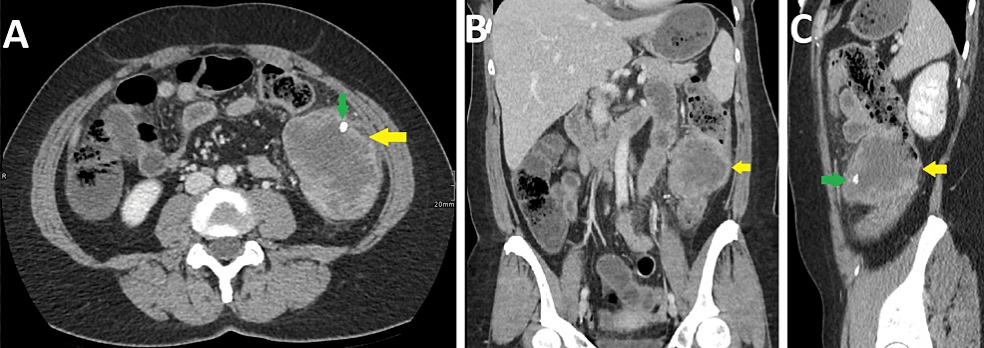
CT abdomen with contrast Axial view (A), Coronal view (B), and Sagittal view (C) show a large necrotic tumor involving the descending colon with an exophytic component (yellow arrow) and calcification within it (green arrow).
